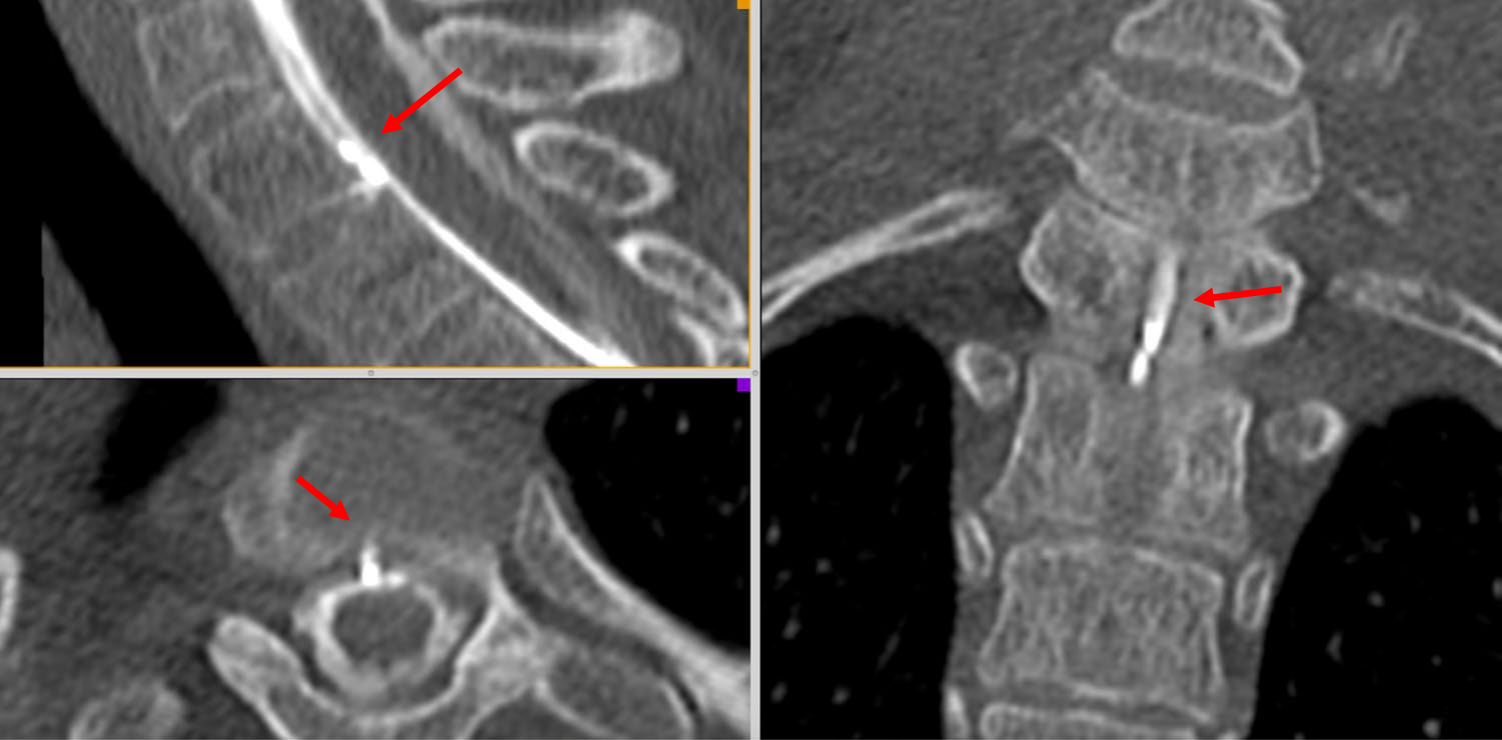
Pre-operative myelo-CT scan showing the ventral dural leak
